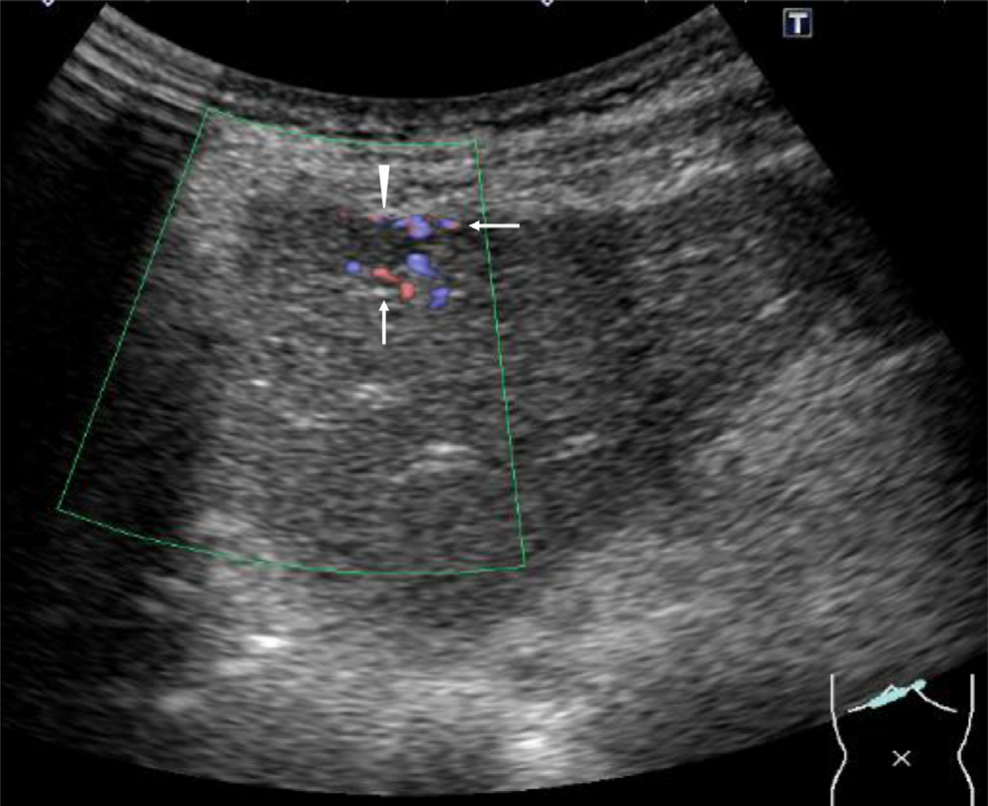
Ultrasonography showed a presumed tumor (arrowhead) with obscured margins and abundant vascularity (arrows).
